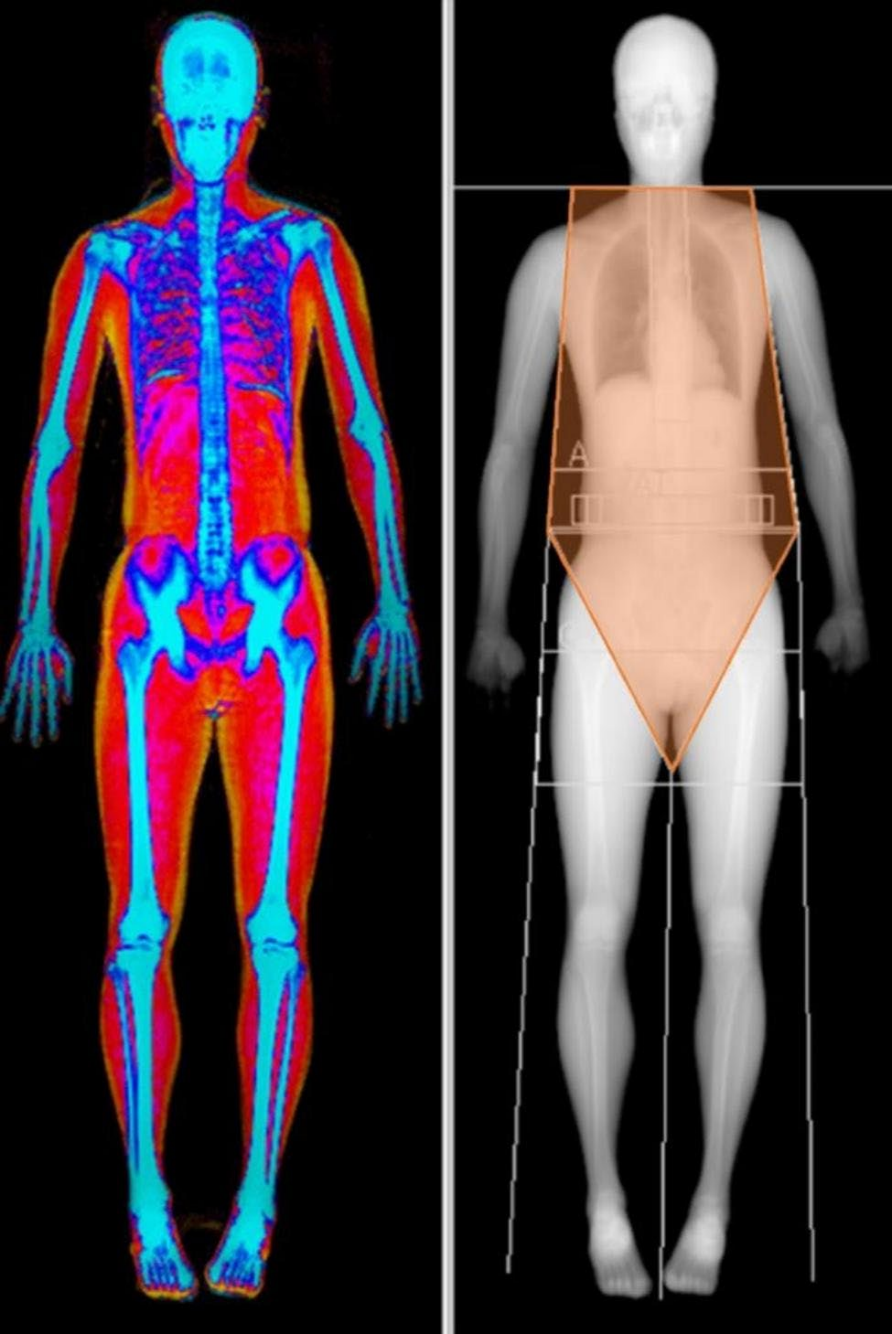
Dual-energy X-ray absorptiometry (DXA) scan with lean mass analysis Colors in the DXA images represent tissue densities, with yellow (fat) being the lowest density and blue (bone) being the highest density. Trunk muscle mass is defined as the trunk fat-free mass, excluding the head and all limbs
